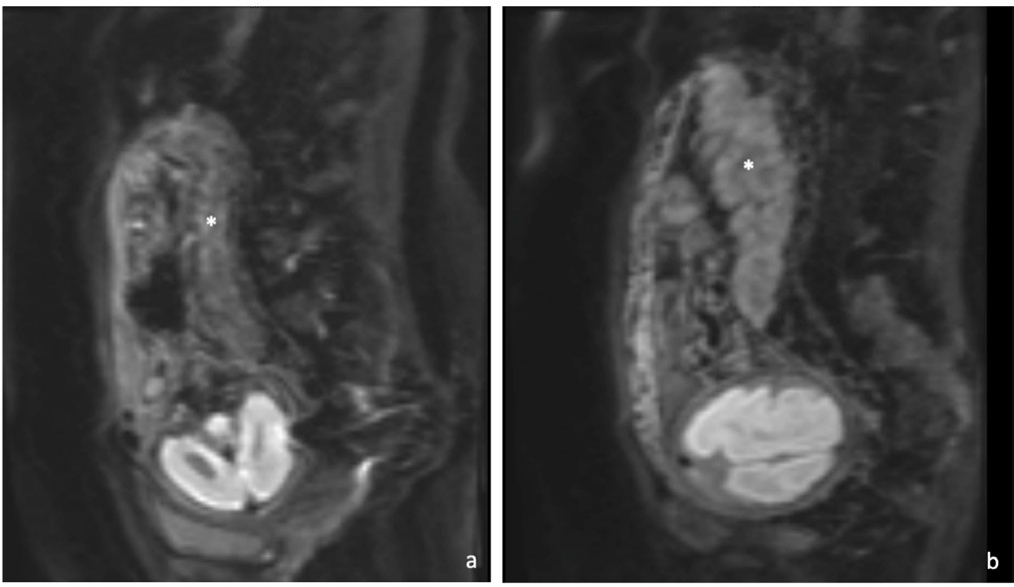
(a) Sagittal DWI MRI showing reduced diffusion in the placenta (*), (b) DWI MRI of normal fetal MRI scan at 29 weeks gestation, the placenta is uniformly bright (*).
